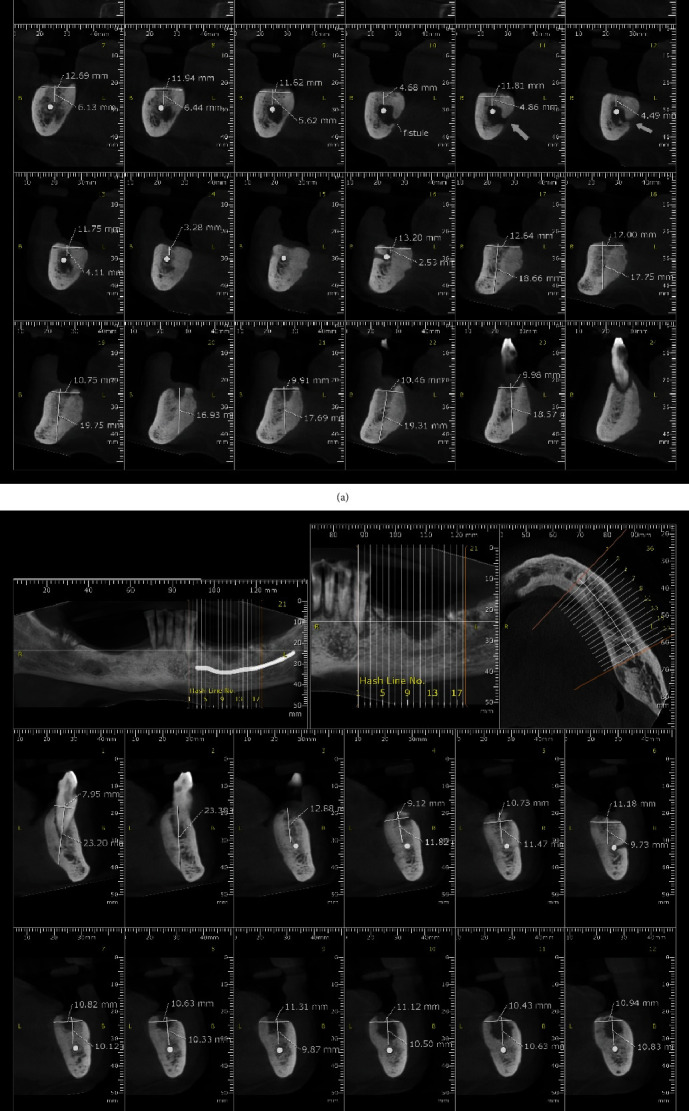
Pre-operative CBCT images of the patient depicting ill-defined patchy lytic radiolucent areas in the mandible surrounded by sclerotic bone in the posterior region on the (a) right and (b) left sides.
